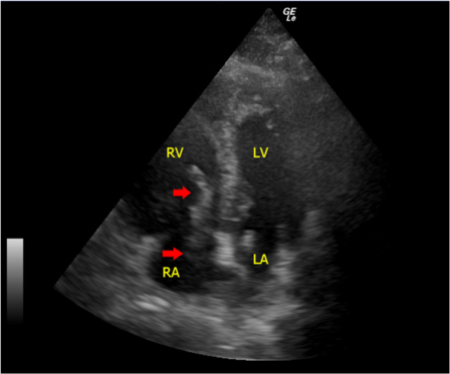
Pulmonary embolism. In this apical four-chamber view, echogenic blood clots (*red arrows*) in the right atrium protruding into the right ventricle through the tricuspid valve during diastole are seen in a patient with confirmed massive pulmonary embolism(*RA* right atrium, *RV* right ventricle, *LA* left atrium, *LV* left ventricle)
